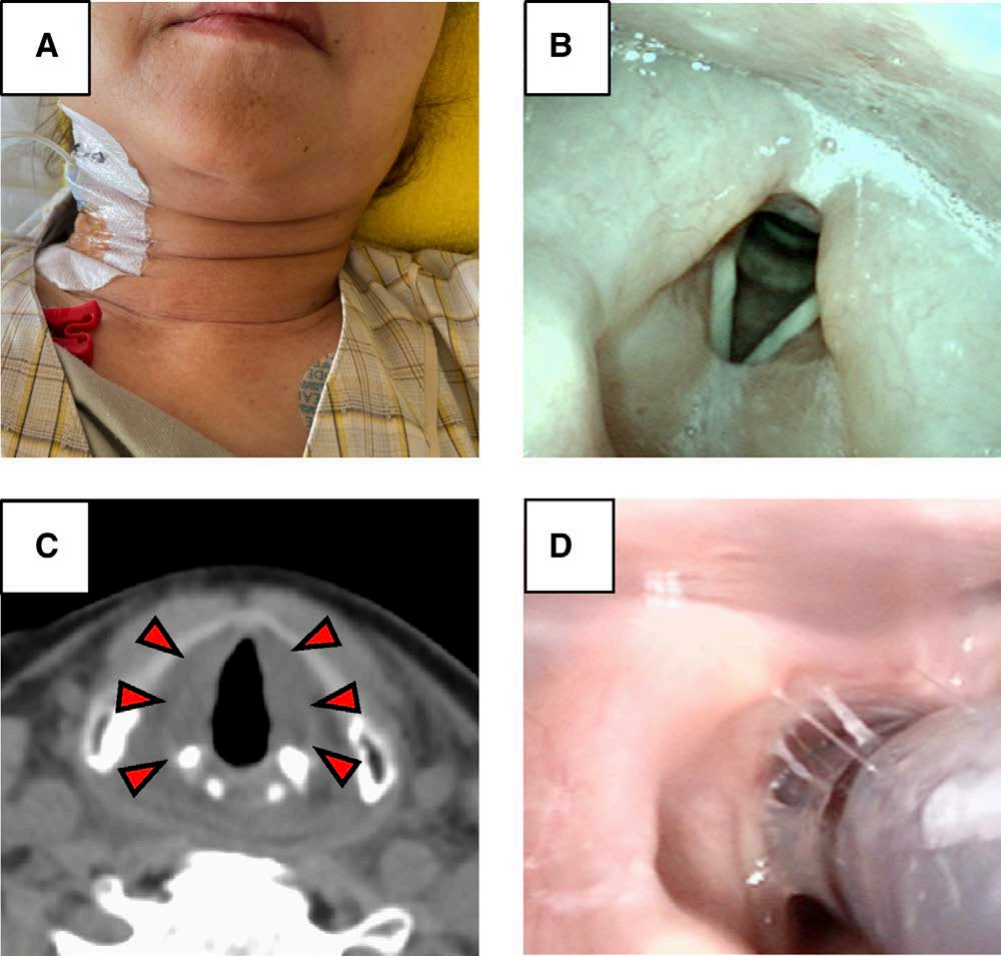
(A) Photograph of the face and neck on day 4 after CAR-T cell administration. Swelling of the face and neck is observed. (B) Image from laryngoscopy. Marked laryngeal edema can be seen. (C) Neck CT before tracheal intubation. Marked laryngeal edema can be seen (red arrows). (D) Image from laryngoscopy. Laryngeal edema is improving.
